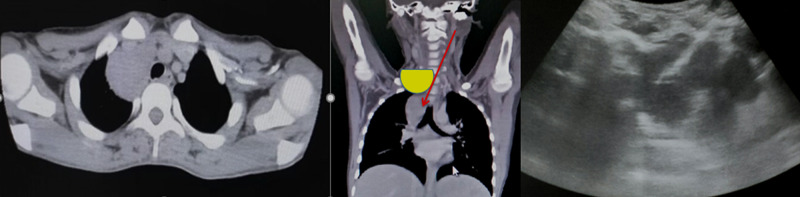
Plain CT scan of the chest showing a large anterior mediastinal mass in the right paratracheal location. Ultrasound-guided para-sternal needle placement was performed for transthoracic mass biopsy.
